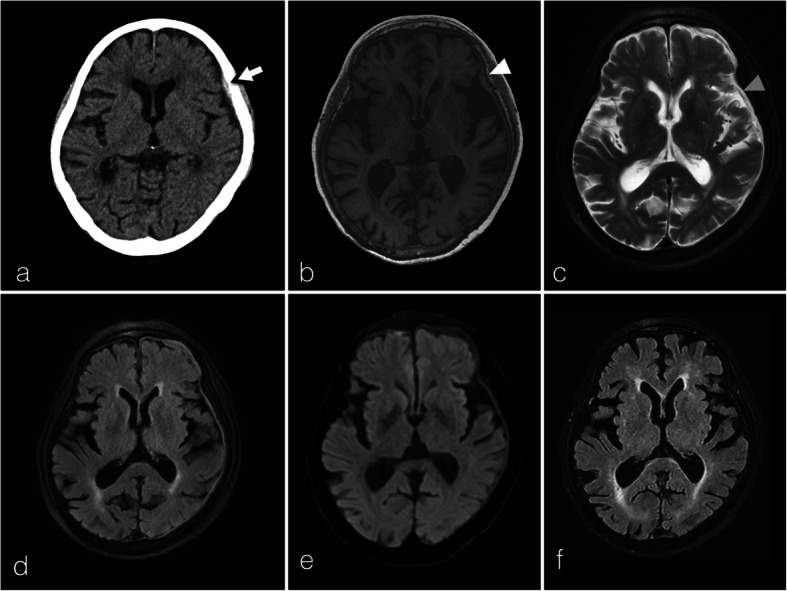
Patient 1. Axial non-contrast-enhanced CT brain scan revealing a left frontal convexity region hyperdense extra-axial lesion resembling an acute subdural hematoma (a, white arrow). A non-contrast-enhanced MRI scan exhibiting a T1-weighted isointense (b, white arrowhead) and T2/FLAIR-weighted hypointense left hemispheric pachymeningeal lesion with areas of hyperintense signal changes representing inflammatory foci (c, T2W, grey arrowhead; d, FLAIR sequence). The lesion did not show evidence of restricted diffusion (e). Three months after initiating prednisolone, there was complete resolution of the pachymeningeal lesion (f, FLAIR sequence)
